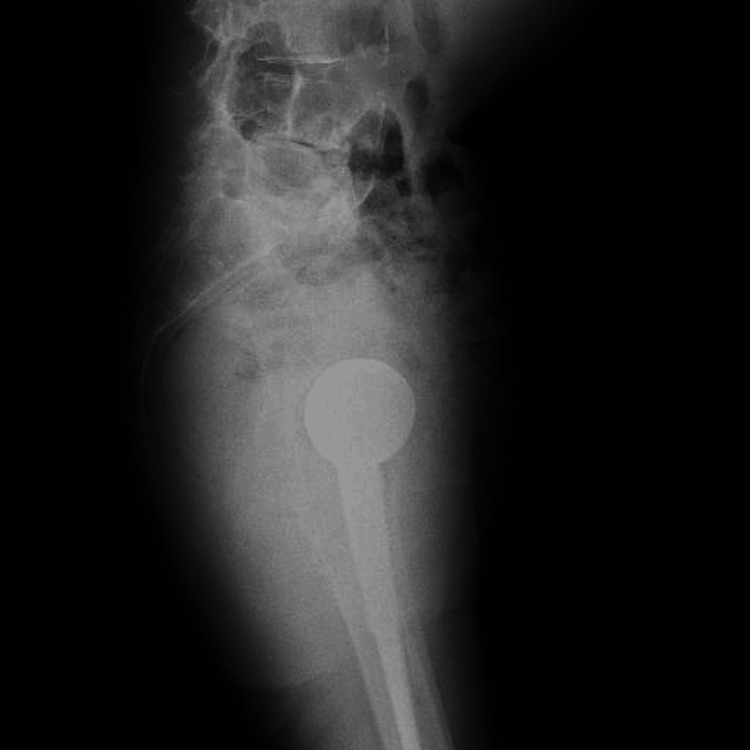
Plain radiograph of the pelvis at admission (lateral view). A right acetabular fracture was identified, with no obvious loosening of the femoral stem.
